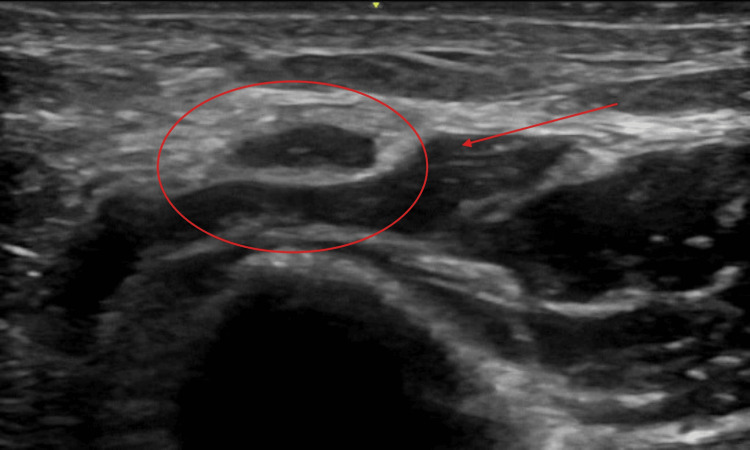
Sonographic evidence of ulnar nerve compression at the cubital tunnel Ultrasound imaging demonstrates focal enlargement of the ulnar nerve within the cubital tunnel, with increased cross-sectional area compared to proximal segments. The nerve appears hypoechoic with loss of the normal fascicular pattern, consistent with compressive neuropathy. Surrounding soft tissue structures contribute to the visible narrowing of the tunnel. Dynamic assessment with elbow flexion further demonstrates nerve subluxation and accentuation of compression, supporting a position-dependent etiology.
